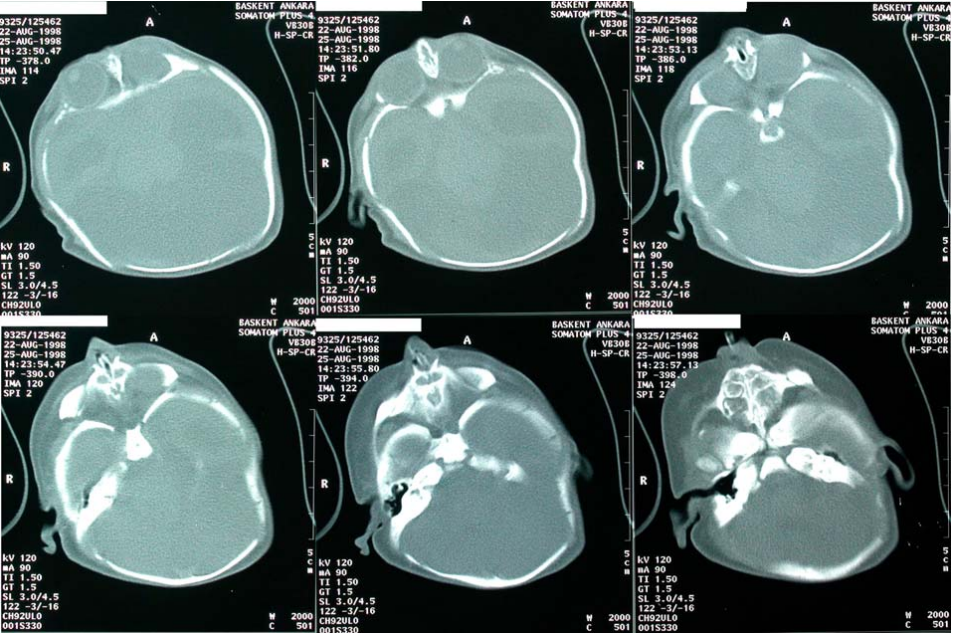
Consecutive axial computed tomography sections from superior to inferior demonstrating absence of bony nasal structures and paranasal sinuses. A catheter is placed inside the single-blind cavity demonstrating a lack of communication with the pharynx.
